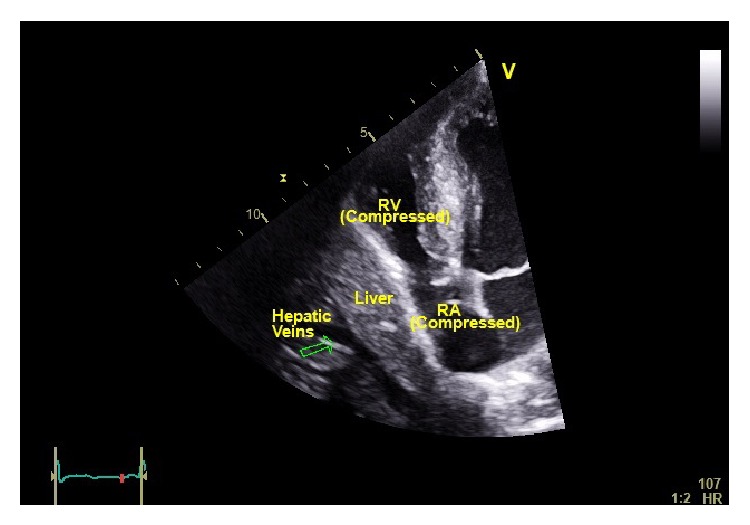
Transthoracic apical four-chamber image obtained after transplant but prior to the diphragm plication. Hepatic compression of the atrium and ventricle is evident here. RV: right ventricle, RA: right atrium.
